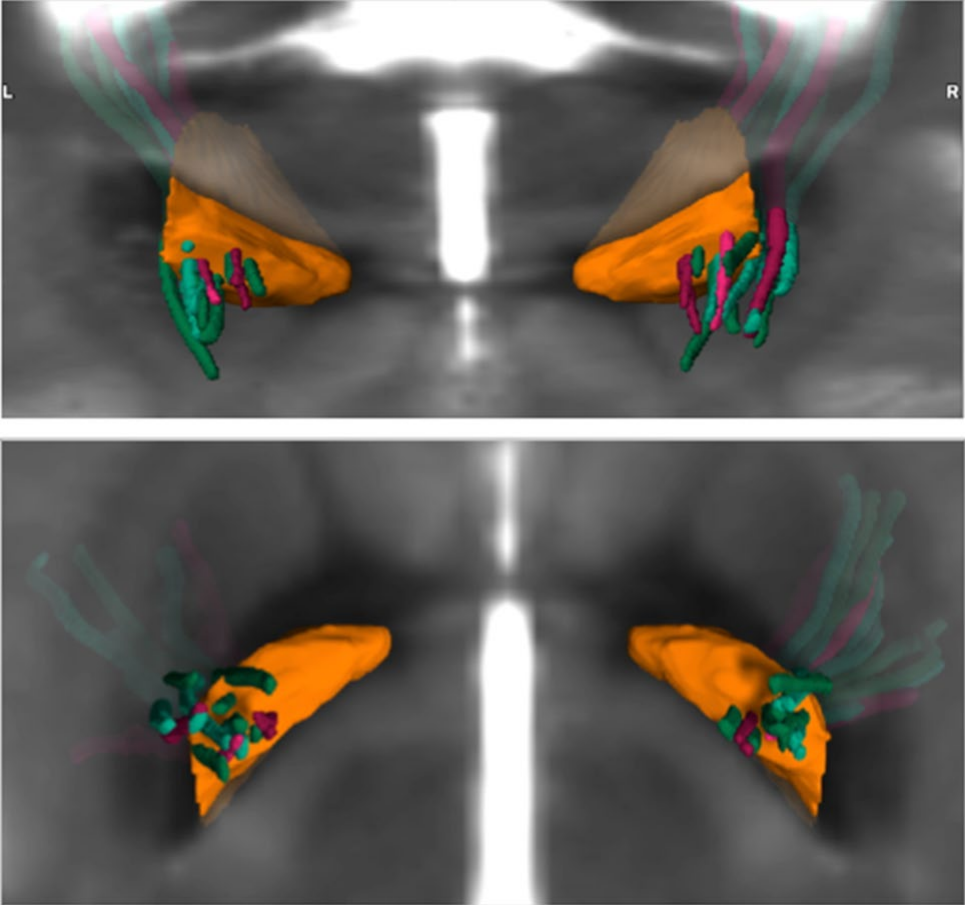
Three-dimensional imaging of electrodes. Three-dimensional imaging of electrodes of responders (green) and non-responders (red) with respect to the GPi (orange) of the Brainlab Atlas displayed on a normalized MRI. As the leads were transferred to the common reference space by elastic fusion, they are not straight but bent. There is no distinct clustering of electrodes of responders vs. non-responders
